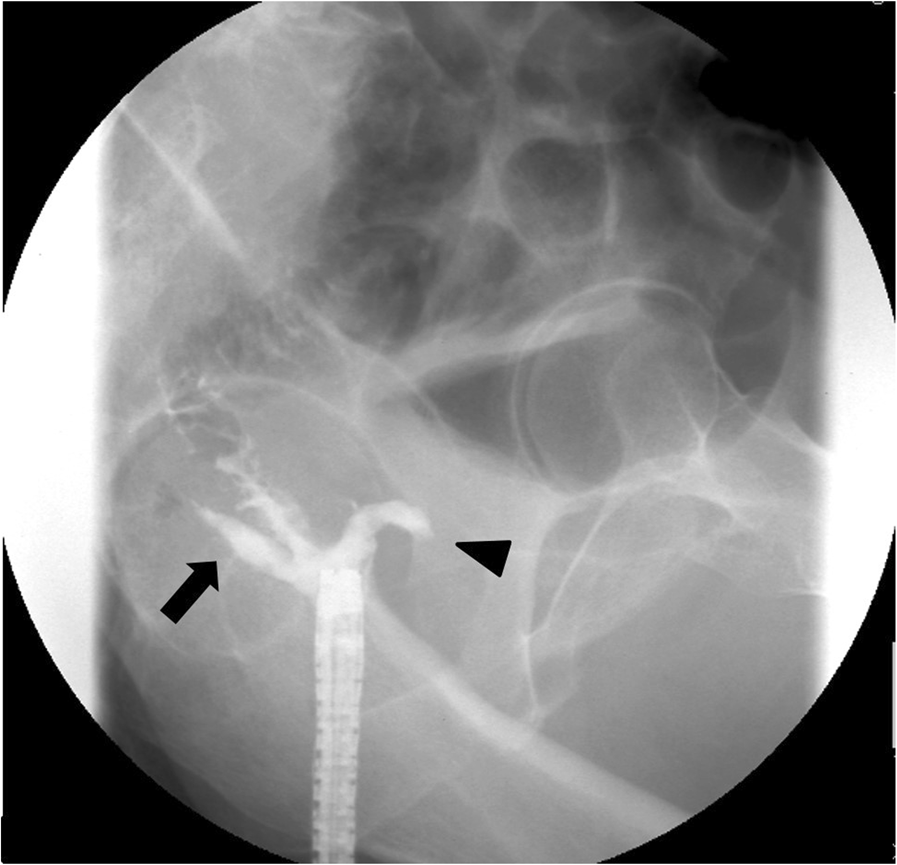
Fistula formation was observed at the anastomotic site after low anterior resection on contrast radiography. Arrow head and arrow indicate fistulae which go to the anterior and posterior side of the anastomosis, respectively. There was no anastomotic-urethral fistula seen on contrast enema
